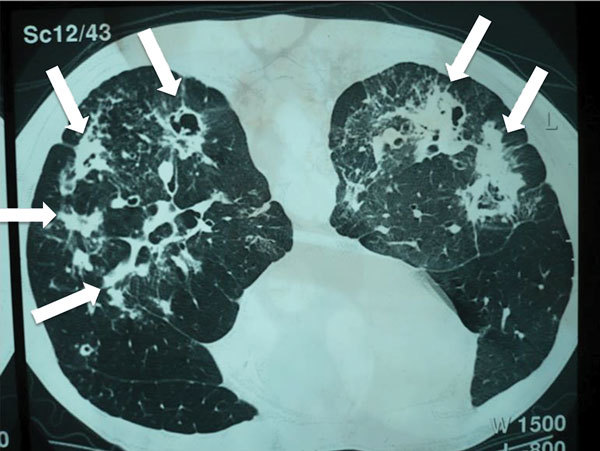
Computed tomography radiograph of thorax showing chronic pulmonary histoplasmosis with bilateral cavitary infiltrates resembling pulmonary tuberculosis, coccidioidomycosis, paracoccidioidomycosis, and aspergillosis. Arrows indicate areas of abnormality. Image used with permission of Arnaldo Colombo (©2016, all rights reserved).
